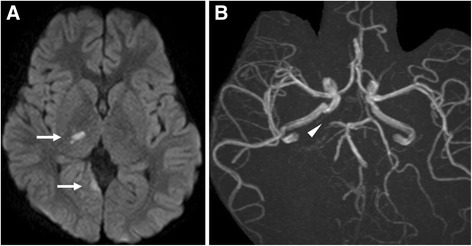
a Magnetic resonance imaging shows ischemic strokes in the right thalamus and right occipital lobe (arrows). b Magnetic resonance angiography shows obstruction of the right posterior cerebral artery (arrowhead)
